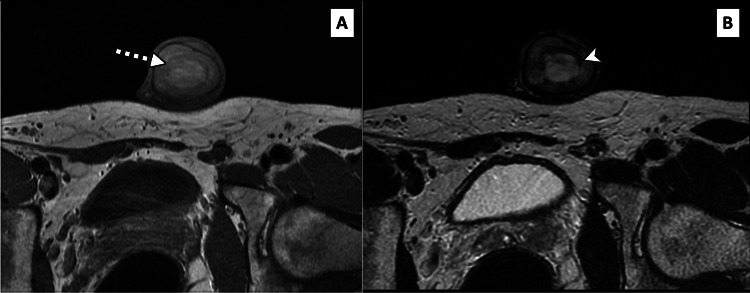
Axial T1-weighted (A) and T2-weighted (B) penile MRI Note how the midline penile collection is both bright on T1 (dashed white arrow) and T2 (white arrowhead), consistent with a penile haematoma.
